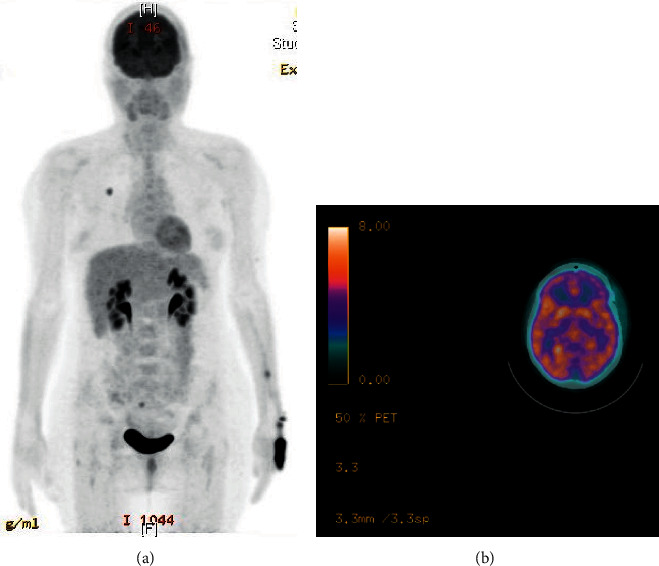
PET scan. Postsurgical changes are present in the left frontal parietal lobe. Punctate hypermetabolic foci are present in both cerebral hemispheres and cerebellum compatible metastatic lesions. There is a 1.2 cm hypermetabolic nodule in the right upper lobe with a max SUV of 4.3.
